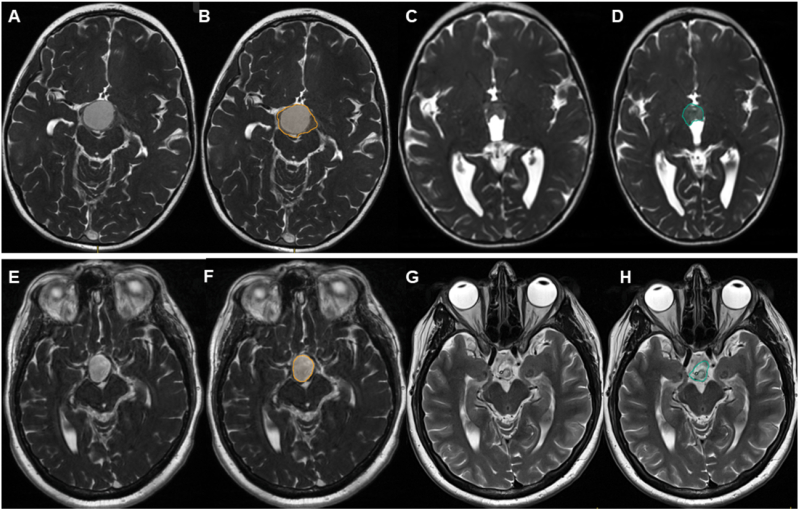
Representative pre- and postoperative CISS MRI scans of predominantly cystic craniopharyngiomas. A–D: Case treated with stereotactic cyst aspiration showing preoperative imaging (A) with corresponding volumetric segmentation (B) and postoperative cyst reduction (C) with segmentation (D). E–H: Case treated with stereotactic internal shunting showing preoperative imaging (E) with segmentation (F) and postoperative cyst reduction (G) with segmentation (H).
